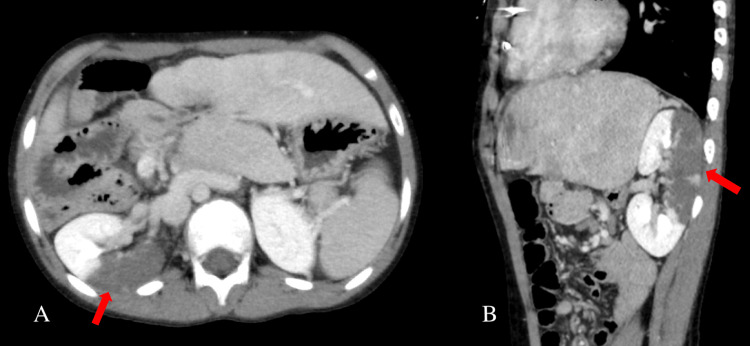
Contrast-enhanced CT of the abdomen at onset shows axial (A) and sagittal (B) views. These images reveal an extensive hypoabsorptive zone on the dorsal surface of the right kidney, with no cortical rim sign. The size of the infarct is 8 cm × 4 cm.
